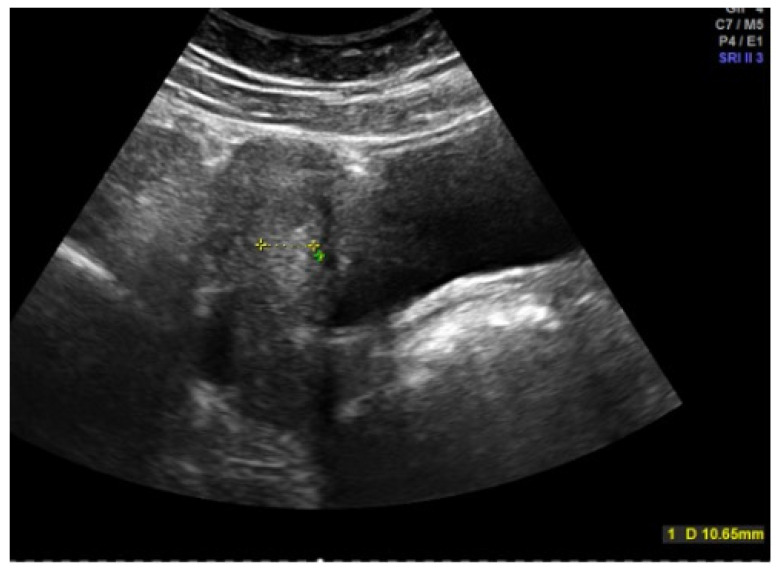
Abdominal sonography: Messurement of Excessively thickened endometrium in a 12-year-old girl with juvenile hypermenorrhea (photo by the authors).
